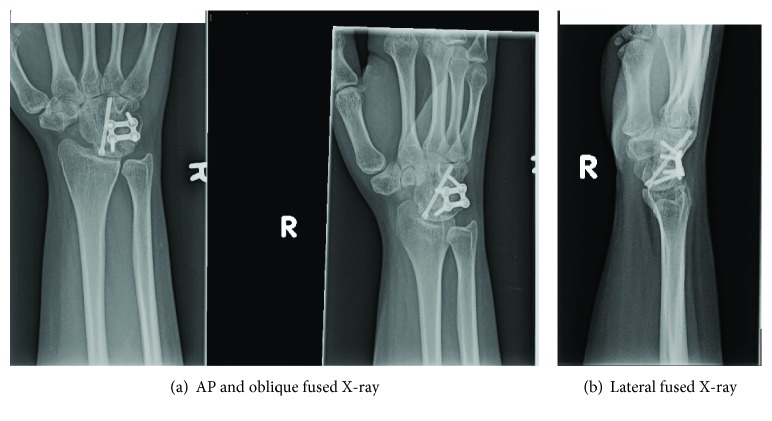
(a, b) AP and oblique (a) and lateral (6) radiographs of the right wrist, demonstrating consolidated 4-bone carpal fusion.
